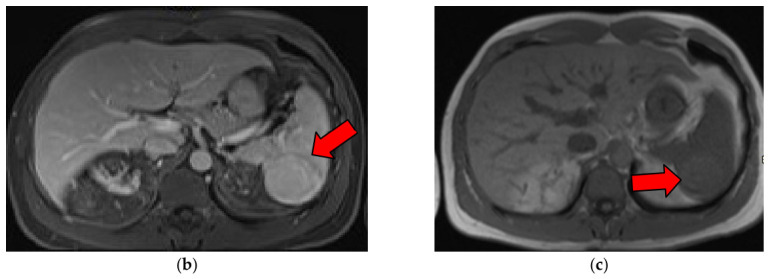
28 year old male with an incidental splenic lesion. (a) Ultrasound shows a mildly hyper-echoic lesion with mild vascularity. (b) On post-gadolinium T1 fat-suppressed imaging, there is an abnormal expansile contour of the mass with signal characteristics similar to background spleen. (c) On an axial pregadolinium T1 imaging, the hamartoma is well circumscribed with minimally increased T1 signal compared to the native spleen.
